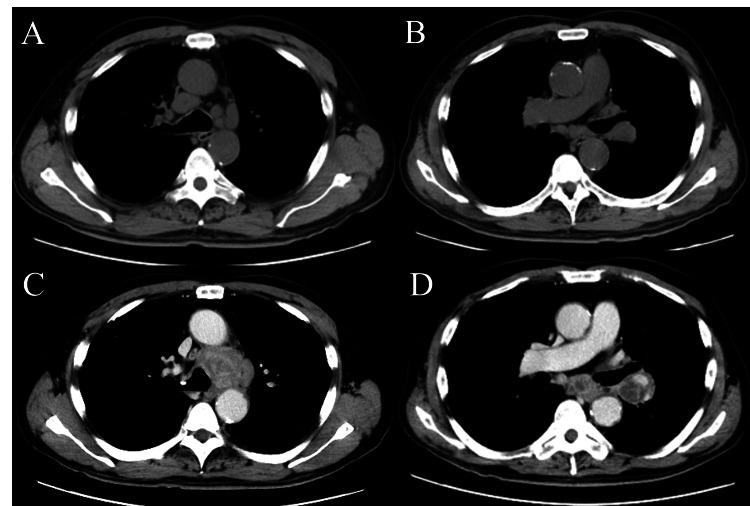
Computed tomography showing enlarged mediastinal lymph nodes at different time points. (A) and (B) were taken 18 months after surgery for the first cancer, while (C) and (D) were captured 51 months post-surgery, coinciding with the diagnosis of the second cancer. Images A and C depict the lower paratracheal region, and B and D show the subcarinal to hilar region.
